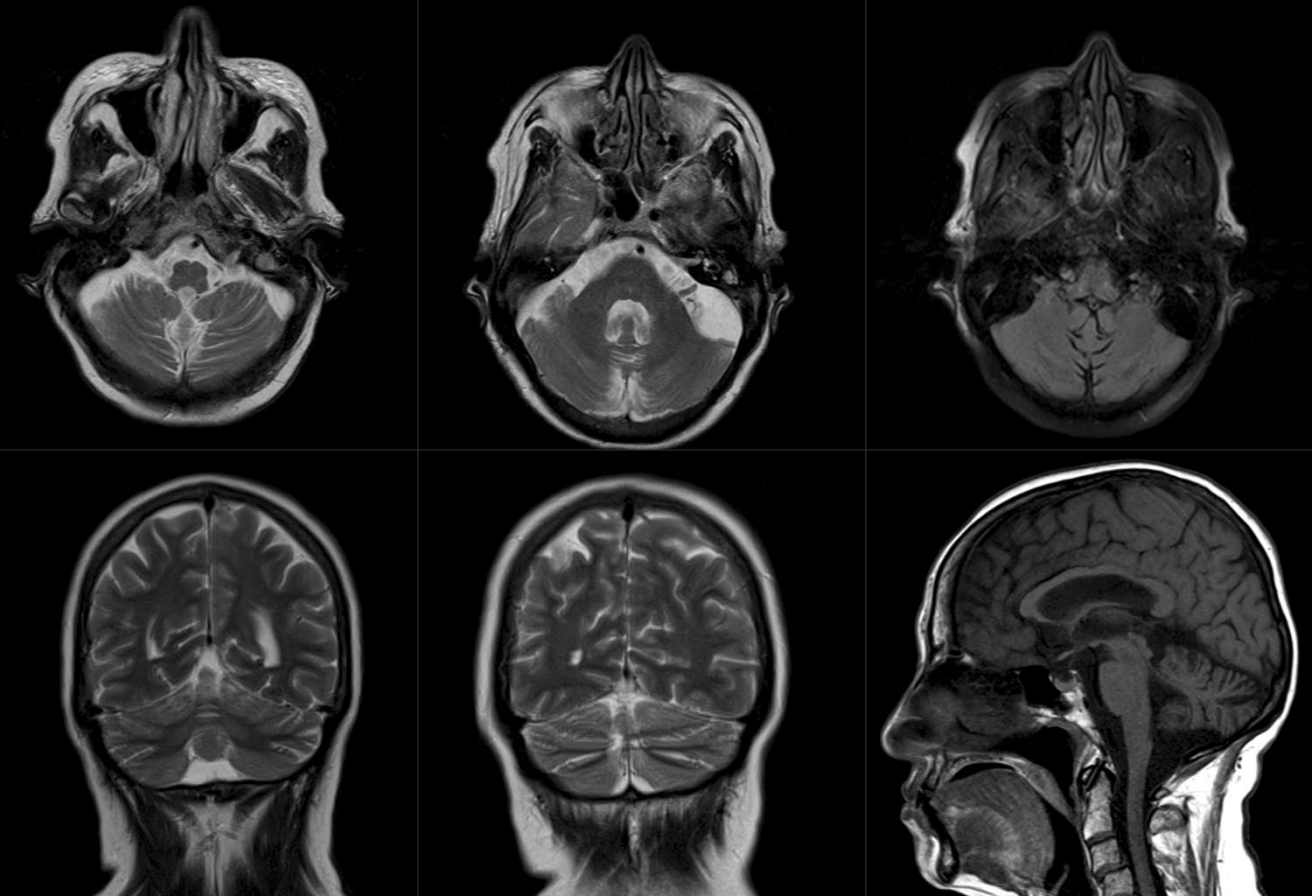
Neuroimaging in a HTLV-1-positive patient with ataxia. Brain magnetic resonance imaging of the reported patient at age 62 years of age showing diffuse subtle atrophy of the cerebellar hemispheres and vermis. Top row: axial T2-weighted (left and middle) and T2-weighted fluid-attenuated inversion recovery (right). Bottom row: coronal T2-weighted (left and middle) and sagittal T1-weighted (right) images
